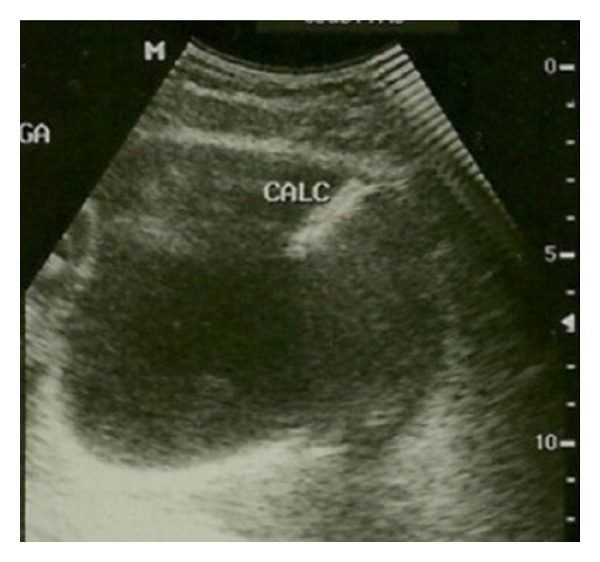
Transabdominal ultrasonography showing a 20 mm calculus located in the bladder dome.
